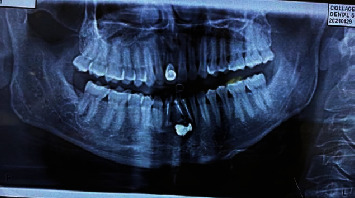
Panoramic radiograph showing mixed radiopaque and radiolucent area in relation to 32-43.
